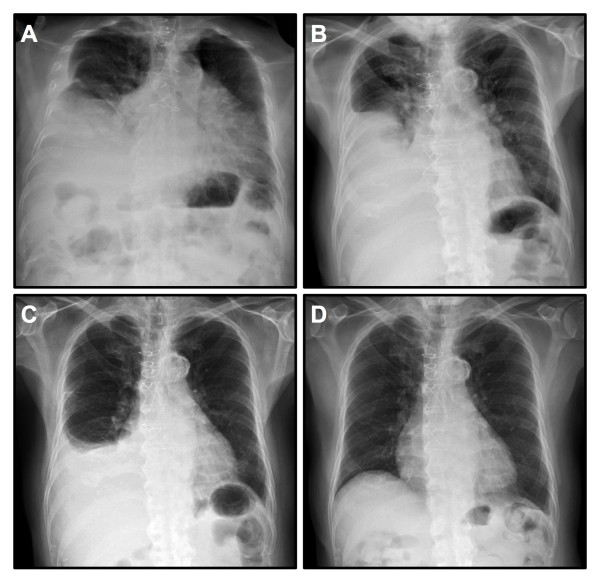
Chest X-ray images. (A) Large pleural effusion in his right lung field found on the first admission. (B) Large pleural effusion in his right lung field on the second admission. (C) Pleural effusion in his right lung field on the current admission, two weeks after the last previous thoracocentesis. (D) Clear chest X-ray six months after the discontinuation of isoniazid.
